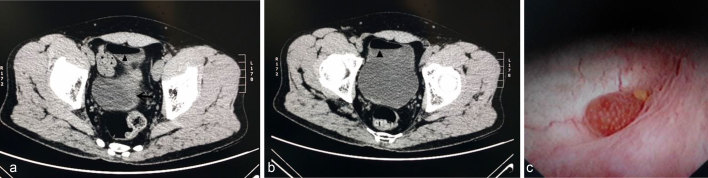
(a) In this case, non-contrast CT scan was performed because of poor health of the patient. (b) The bladder appeared to be thickened, with gas (triangle) visible within it. (c) Cystoscopy demonstrated a “strawberry-like” tumour (size, 0.3 cm) on the left aspect of the posterior wall of the bladder, surrounded by mucosal folds.
